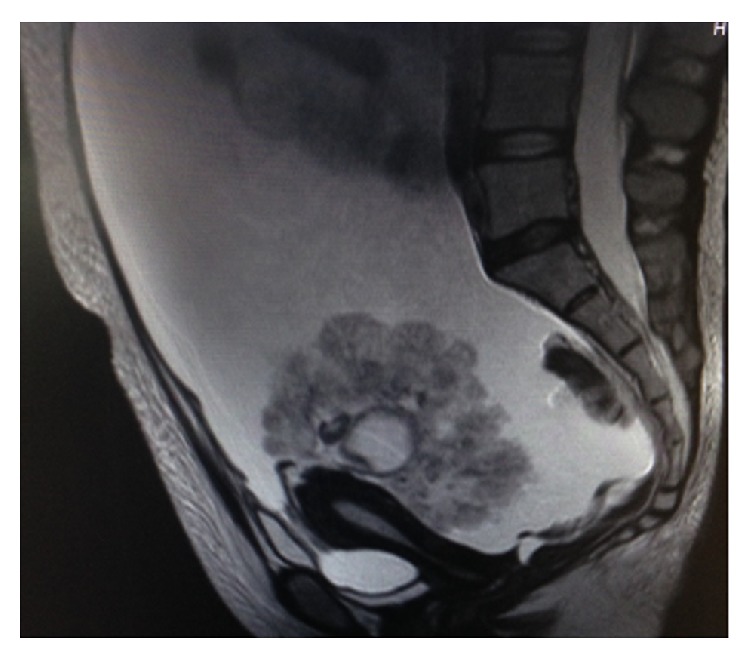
MRI image of patient 1 before operation.
